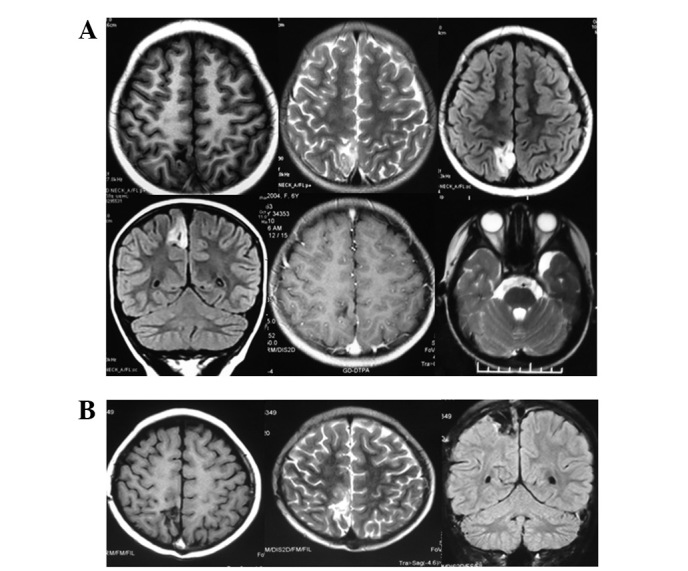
(A) Preoperative T1-weighted image (WI) showed a low signal, while fluid-attenuated inversion recovery (FLAIR) scanning showed a high signal and enhanced scanning revealed no signals. An arachnoid cyst was visible in the left temporal region. (B) At the six-month postoperative review there was no tumor recurrence.
